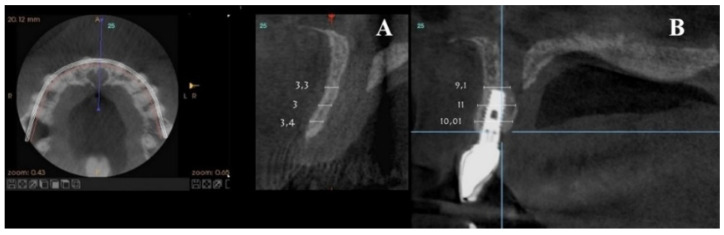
Radiographic control of Patient 1 at their 9-year follow-up. An occlusal view is shown on the left. (A) Sagittal view of the nasopalatine duct prior to treatment. (B) Post-treatment control radiograph: sagittal view of the implant in a three-dimensional position with the regenerated palatal wall.
